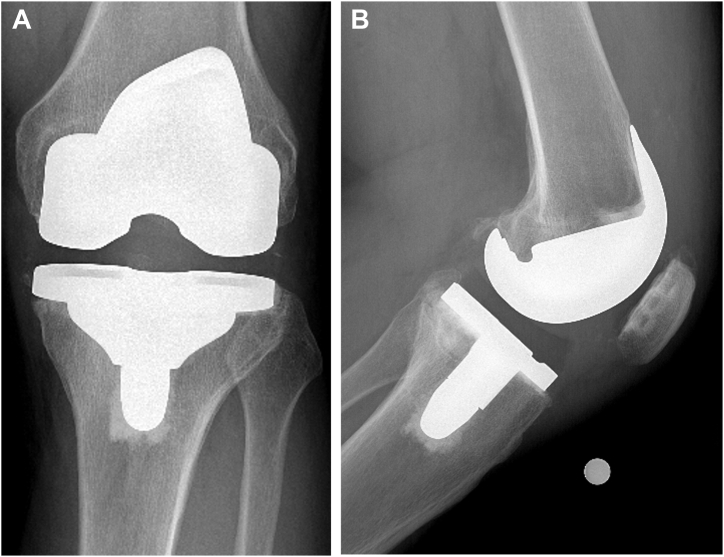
Final anteroposterior (a) and lateral (b) radiographs of a left conversion total knee arthroplasty for a failed unicompartmental knee arthroplasty at 1 year postoperative.
